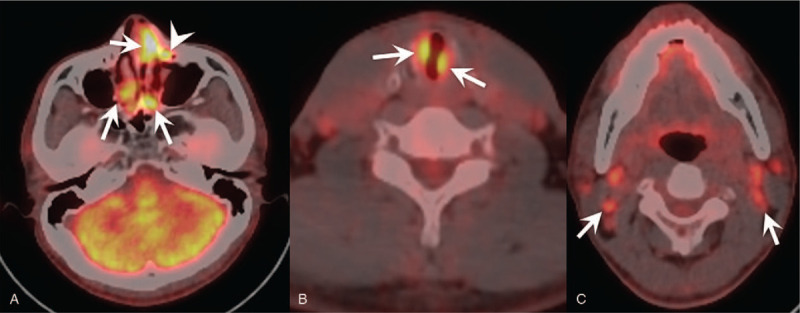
F-18 FDG PET/CT images. (A-C) There are multifocal hypermetabolic lesions in both nasal cavities (arrows on A), left nasolacrimal duct (arrowhead on A), both sides of the subglottic larynx (arrows on B), both cervical lymph nodes (arrows on C).
